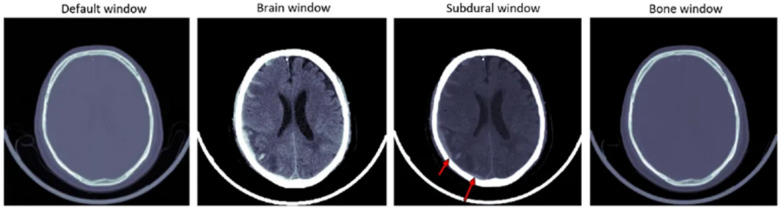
CT scan images under different window settings: default window, brain window, subdural window, and bone window. Images derived from the RSNA Intracranial Hemorrhage CT Dataset (https://www.rsna.org/artificial-intelligence/ai-image-challenge).
